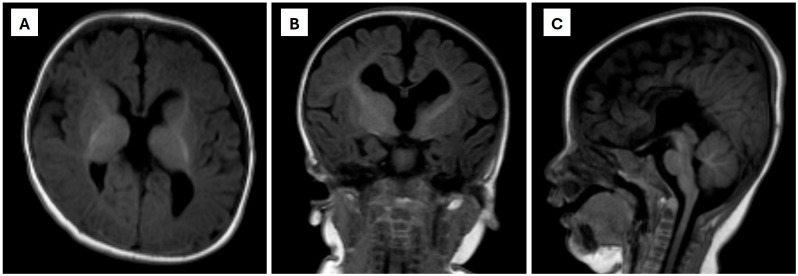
Brain and pituitary magnetic resonance imaging (MRI) findings. T1-weighted axial (A), coronal (B), and sagittal (C) MRI brain images obtained at six months of age show complete agenesis of the corpus callosum, characterized by parallel orientation of the lateral ventricles and colpocephaly. The pituitary gland appears normal on sagittal view.
